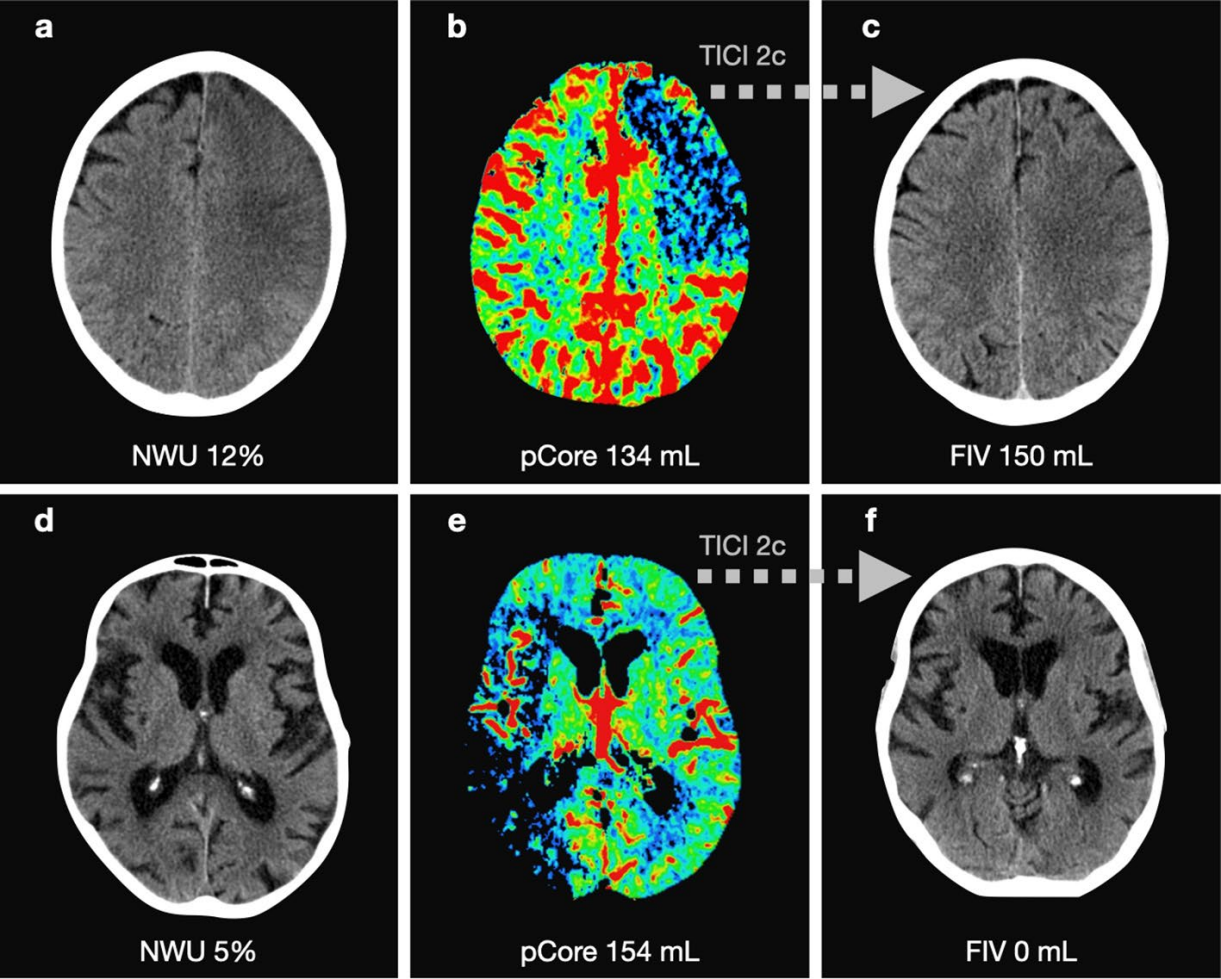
Examples of patients with high (A–C) and low (D–F) NWU and their association with pCore overestimation. Both patients presented within a similar time frame and achieved near-completed reperfusion. (A, D: admission imaging; B, E: rCBF < 20% CTP maps; C, F: follow-up imaging). *NWU* net water uptake; *pCore* rCBF20%-defined core at admission; *CTP* CT perfusion.
